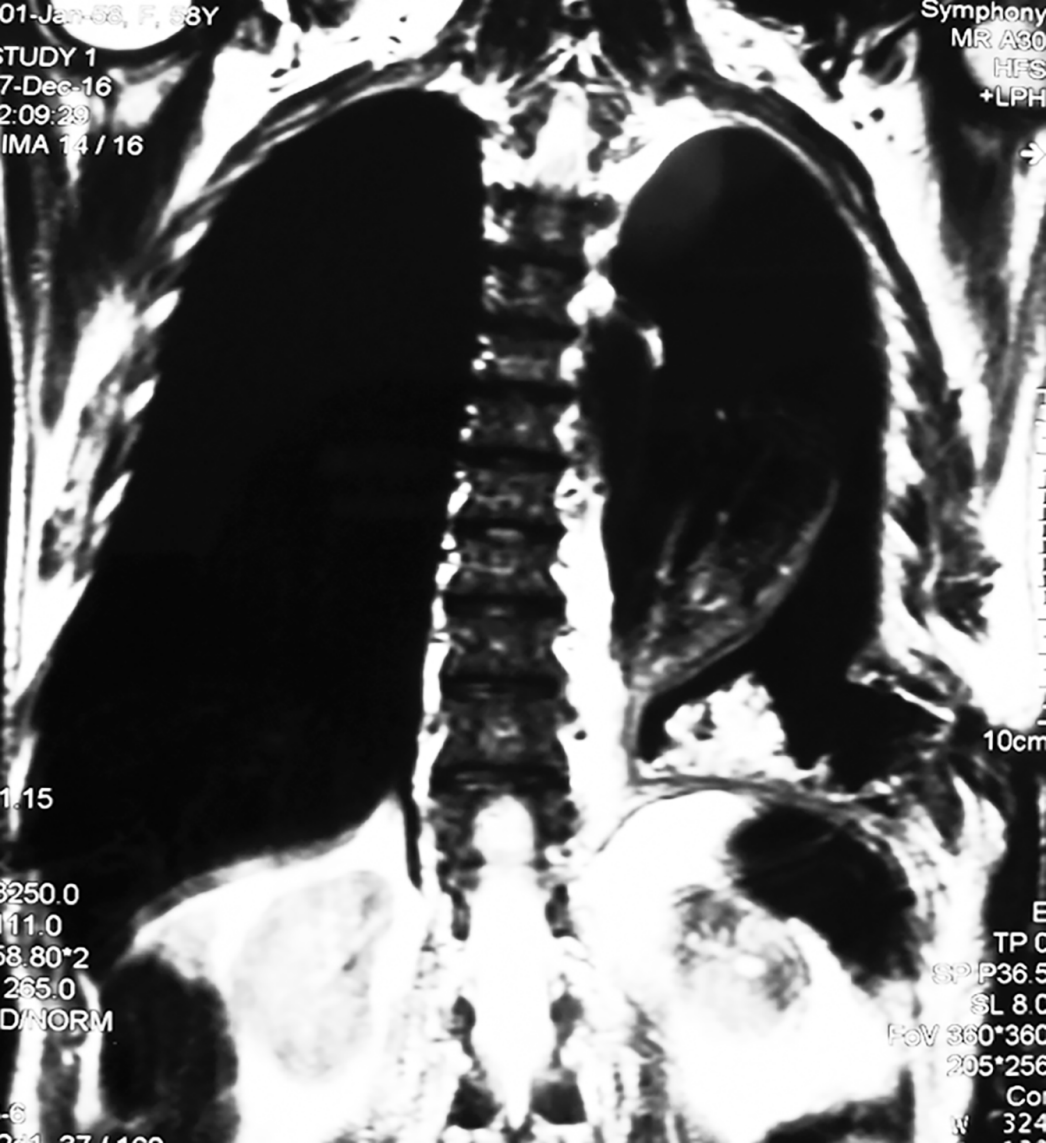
Chest MRI showing marked volume loss in left hemithorax, pneumothorax, soft tissue lesion in the left lingular region, left lower lobe atelectasis, and a small amount of pleural effusion.
